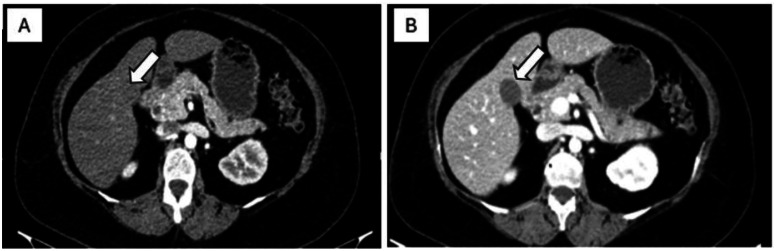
Abdominopelvic contrast-enhanced CT scan images of the patient with postcholecystectomy lesion. Postcontrast abdominal CT scan image shows non enhancing lesion with low density in the bed of cholecystectomy (white arrows). A hypodense lesion in postcontrast arterial (A), and venous (B)phase CT scan image.
